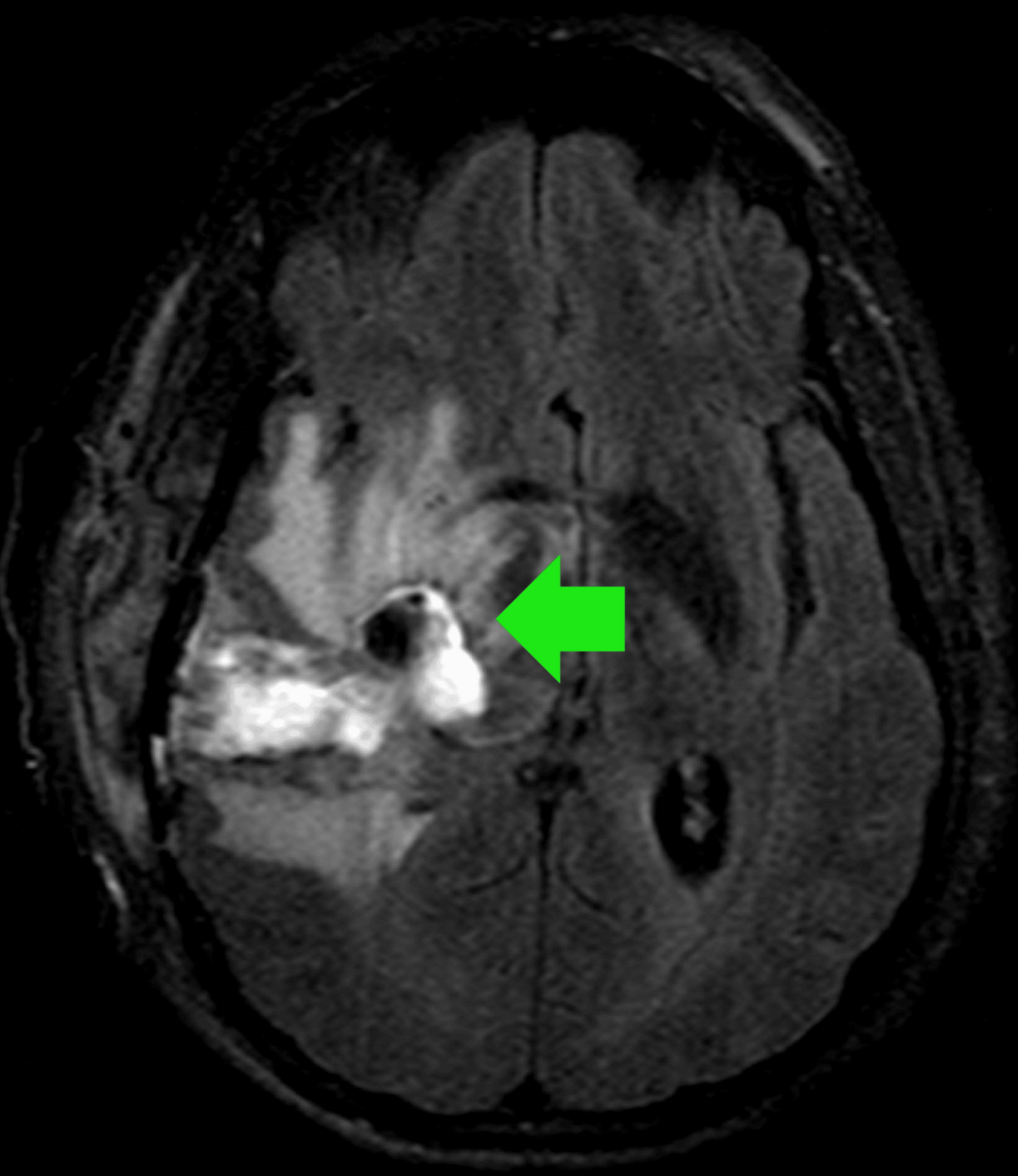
T2/FLAIR MRI of the brain in patient 3 with RPCNSL A heterogeneous mass is present in the medial temporal lobe, with surrounding vasogenic edema. RPCNSL = recurrent central nervous system lymphoma
